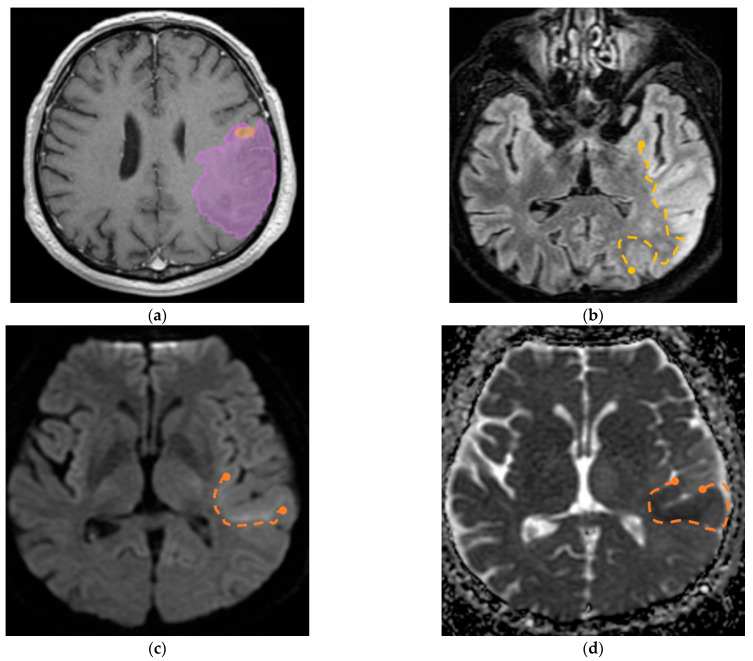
(a) Mdbrain processing of MRI brain acquisition (DICOM) (Scale 1:20)—purple area corresponding to T2W FLAIR hyperintensity; (b) T2W FLAIR (Scale 1:16), axial plane, manual delimitated (yellow dotted line) hyperintense area corresponding to edema/parenchymal invasion; (c) DWI (Scale 1:25), axial plane, discreet hyperintensity in the immediate subcortical area, manually delineated (orange dotted line); (d) ADC map (Scale 1:26)—hypo-intensity corresponding to the DWI hypersignal, suggesting parenchymal invasion, manually delimitated (orange dotted line) (approval was obtained from the Ethics Committee of the University of Medicine and Pharmacy “Grigore T. Popa” Iasi).
